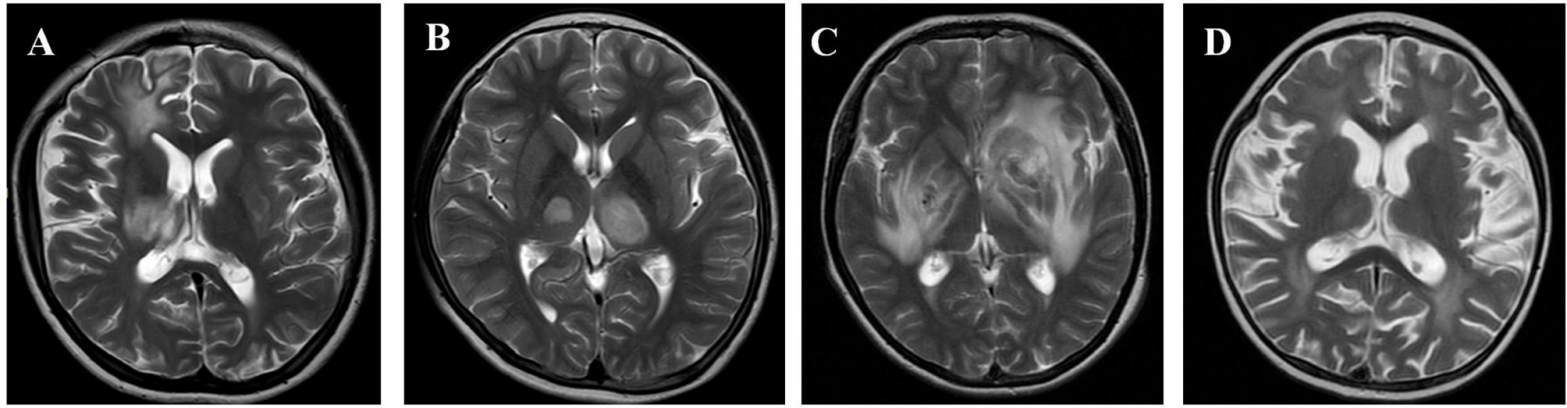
Cranial magnetic resonance imaging of patients. (A) Patient P1: Multiple abnormal bilateral signals in the brain. Both old and new lesions coexist. Demyelinating lesions may be present. (B) Patient P2: Sparse abnormal bilateral signals in the brain parenchyma, suggestive of inflammatory lesions. (C) Patient P3: Abnormal signals in the brain and cerebellum, particularly in the bilateral basal ganglia and the left frontal lobe, suggestive of infective lesions. (D) Patient P15: Abnormal, bilateral symmetrical signals in the white matter, brain atrophy, and similar signals in the thalamus.
